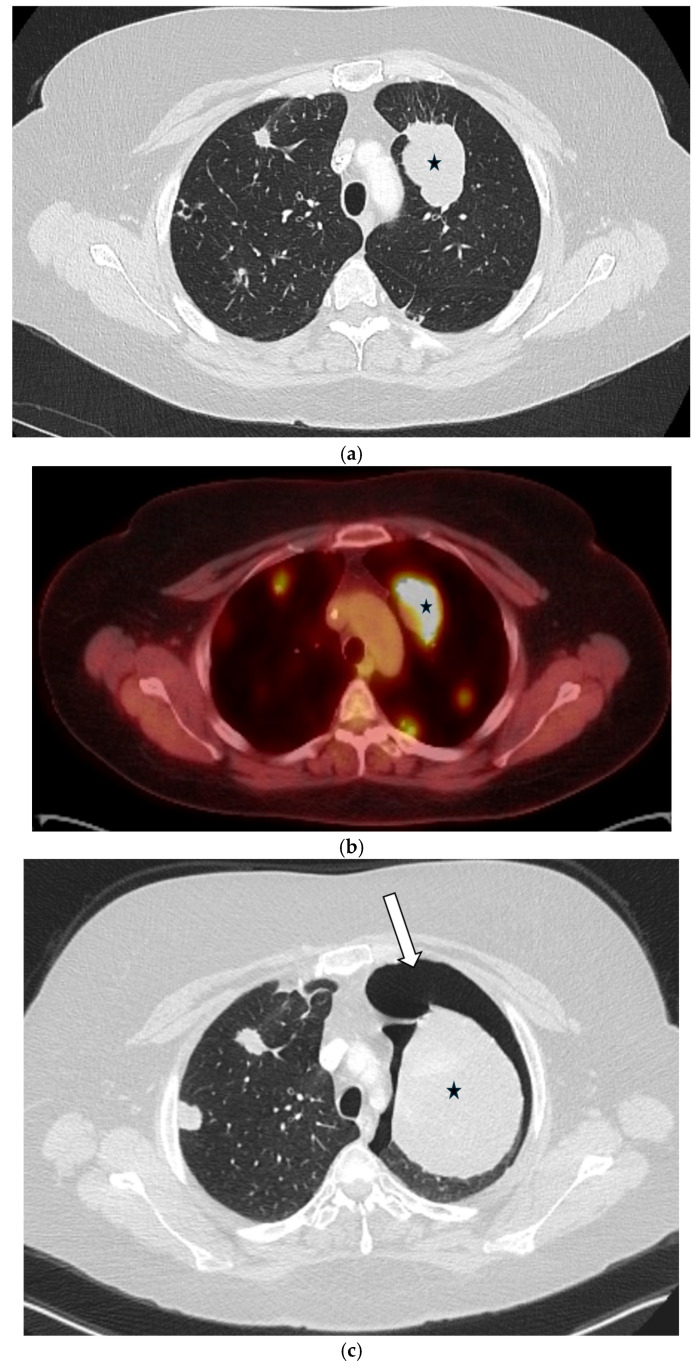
45-year-old female with a history of metastatic rhabdosarcoma. Baseline axial CT image in lung window (a) demonstrates multiple bilateral solid lung nodules and masses (black star) due to metastases. Baseline FDG-PET/CT image (b) shows avid radiotracer uptake (black star) in lung metastases. Subsequent axial CT image in lung window performed 2 months later (c) demonstrates interval worsening of metastatic disease (black star) with new small left pneumothorax (white arrow).
